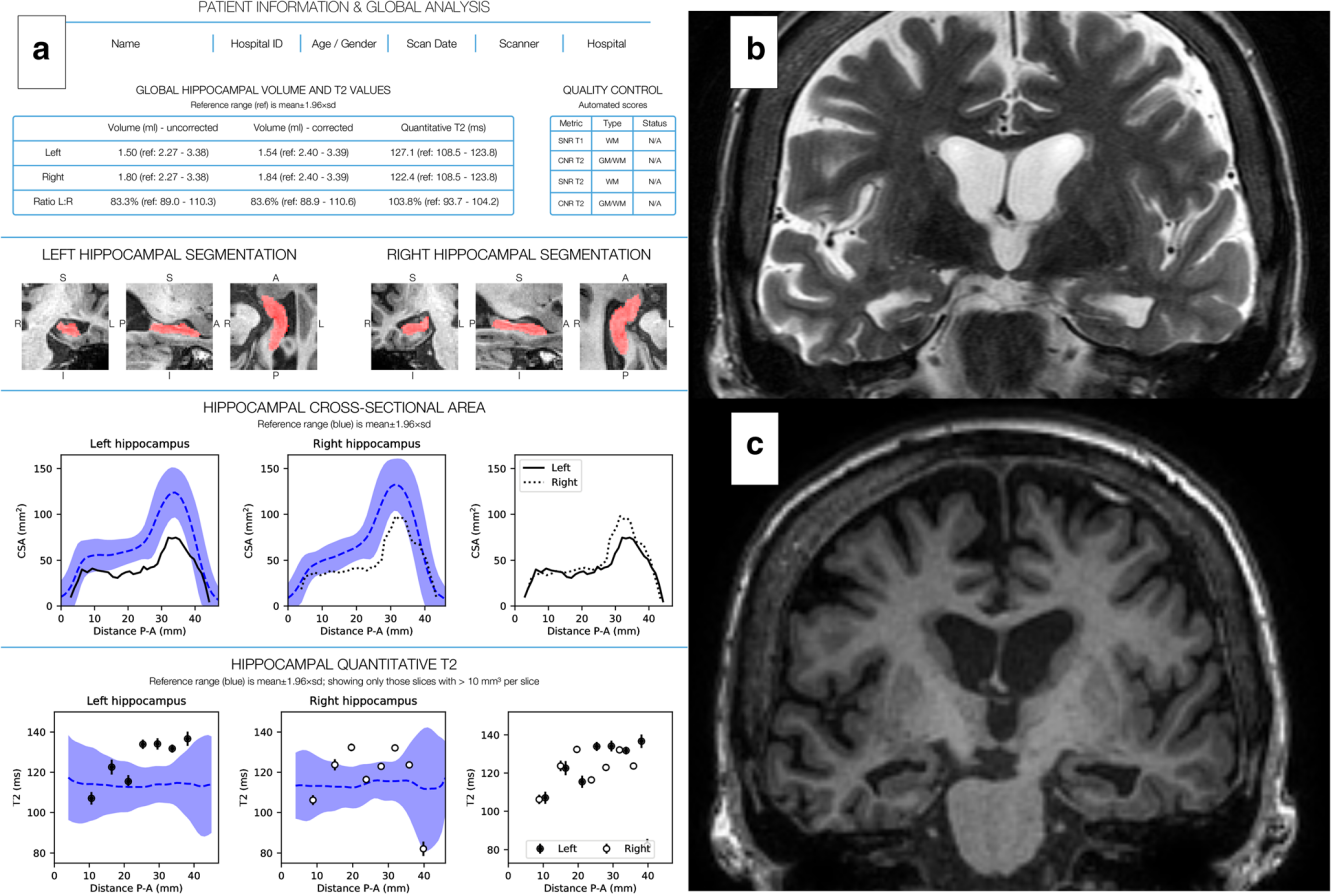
QReport and MR images of a patient with bilateral HS. a QReport displaying patient information; global analysis including global measurements and left:right ratios with normative reference ranges in brackets; quality control; snapshots of hippocampal segmentations; graphs for hippocampal cross-sectional area and qT2 posterior-anterior (P-A) along the hippocampal long axis. Graphical display: black lines or dots represent patient’s values, blue dotted line and blue band represent normative data mean ± 1.96SD, graphs with no reference data are a representation of the patient’s left:right ratio. b Coronal T2 image. c Coronal T1-weighted image
